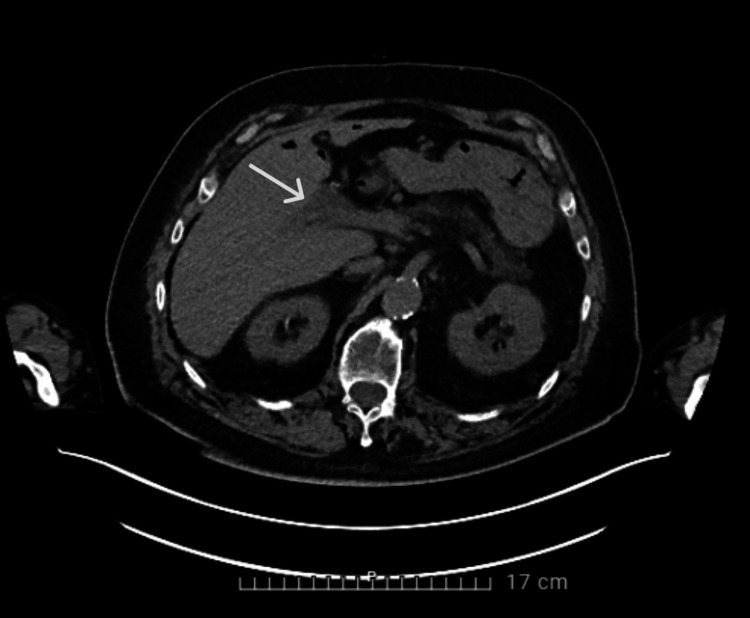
CT scan of the abdomen showing thrombophlebitis of the portal vein
